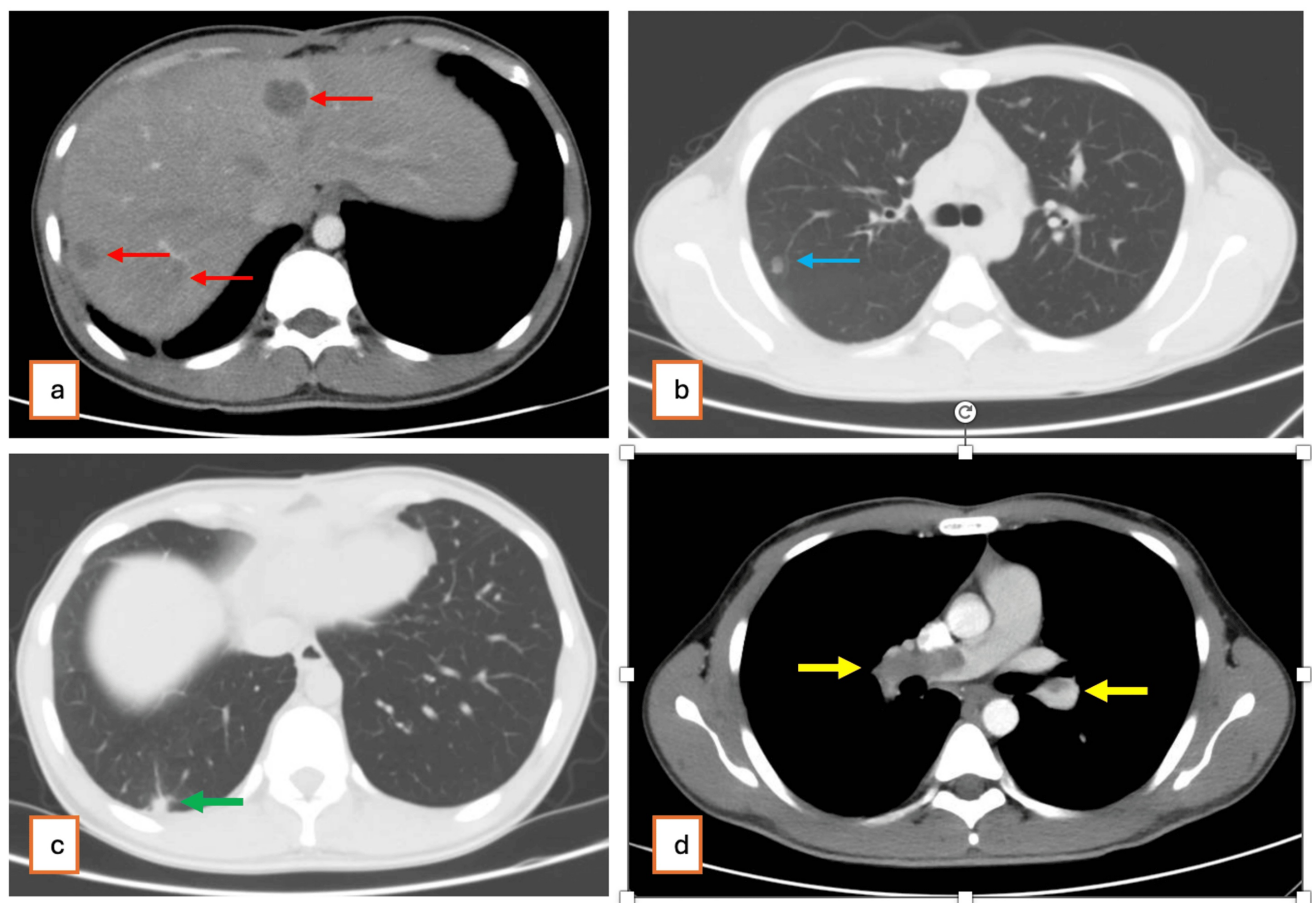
Contrast-enhanced MSCT at three months after surgery. (a) Axial portal venous phase CT shows multiple hypodense lesions in both hepatic lobes (red arrows), consistent with secondary liver metastases. (b) Axial chest CT demonstrates a new pulmonary nodule in the right lung (blue arrow), suspicious for metastatic disease. (c) Axial chest CT shows a pulmonary lesion (green arrow) that was present on the preoperative scan, with no significant change in size or morphology, suggesting stability. (d) Arterial phase imaging demonstrates partial occlusive thrombosis of both the right and left main pulmonary arteries (yellow arrows). MSCT: multislice computed tomography.
